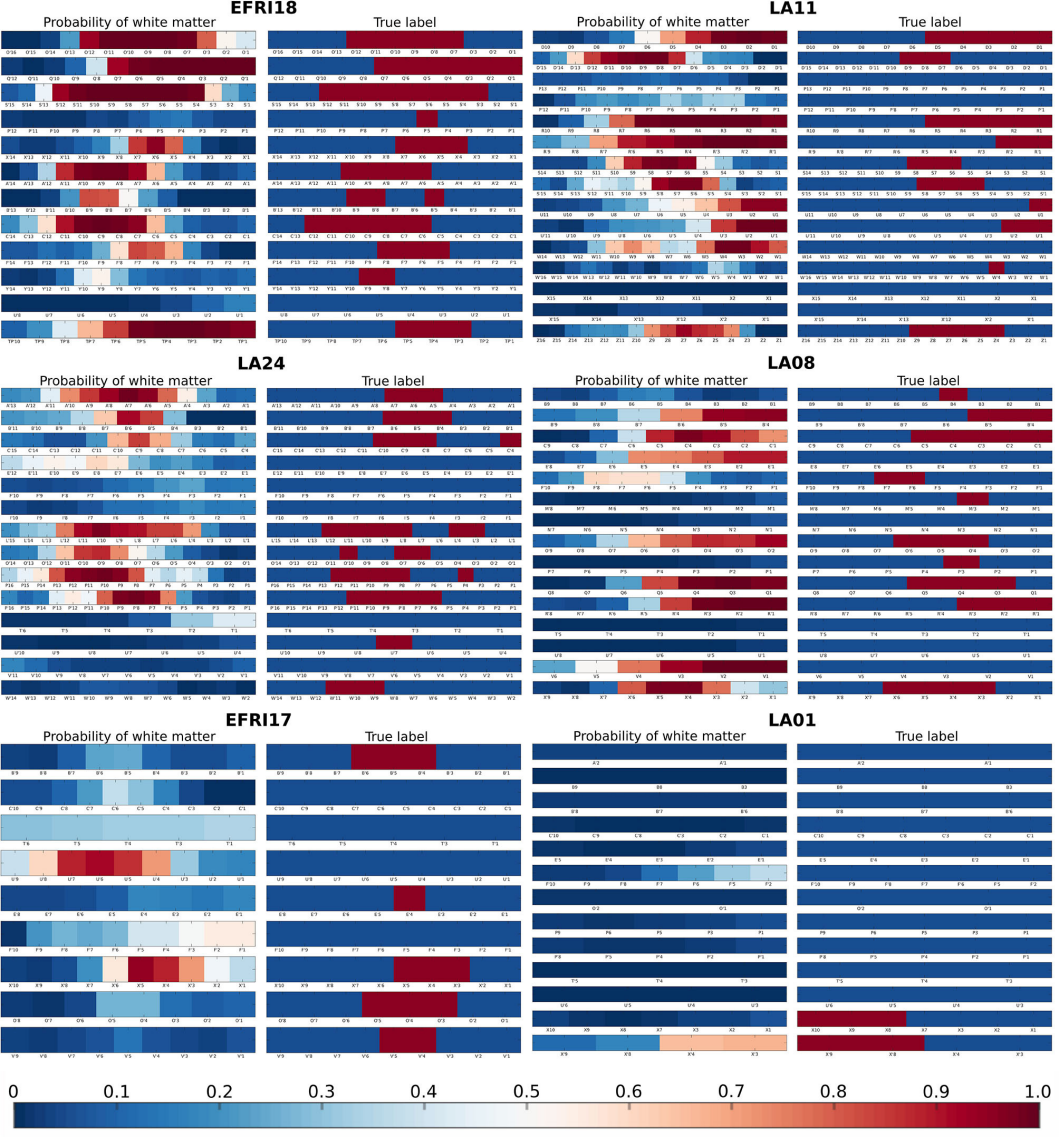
Examples of estimated white matter probabilities for several patients. For each patient, the left column is the probabilities estimated by our classifier, while the right column is the reference labeling done by clinicians using MRI+CT. Each row represents one electrode shank, with individual contacts labeled below. In the reference labeling, red indicates that the contact was labeled as white matter, blue indicates gray matter. In the estimated probabilities, red indicates higher probability of a contact being in white matter according to our classifier. First row: Two of the best patients (EFRI18, LA11), each with > 0.9 AUC. Second row: Two median patients (LA24, LA08), each with 0.86 AUC. Third row: The two worst patients (EFRI17, LA01), representing 0.65 and 0.62 AUC, respectively.
